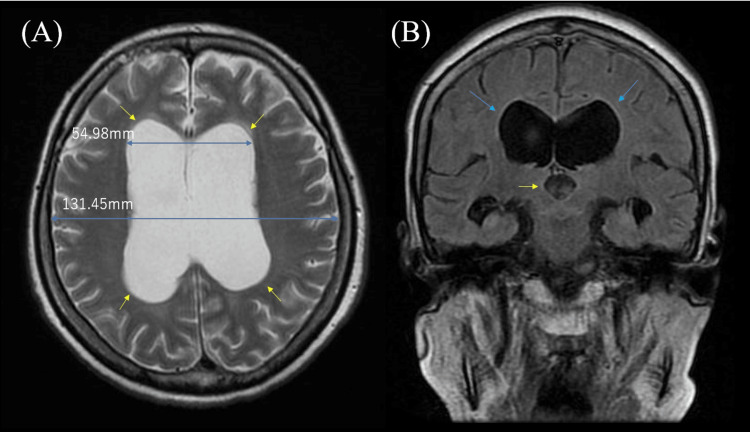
The results of brain MRI. (A) T2-weighted brain MRI showed enlarged lateral ventricles (yellow arrowheads). Evans index (the ratio of the maximum width of the frontal horns of the lateral ventricles and the maximal internal diameter of the skull; blue two-way arrows) was 0.42 (normal-pressure hydrocephalus (NPH) criterion; >0.3). (B) The T2-weighted fluid-attenuated inversion recovery (FLAIR) brain MRI showed enlarged lateral ventricles (blue arrowheads) and third ventricle (yellow arrowhead), but the callosal angle was not narrowed. This patient did not present disproportionately enlarged subarachnoid-space hydrocephalus.
